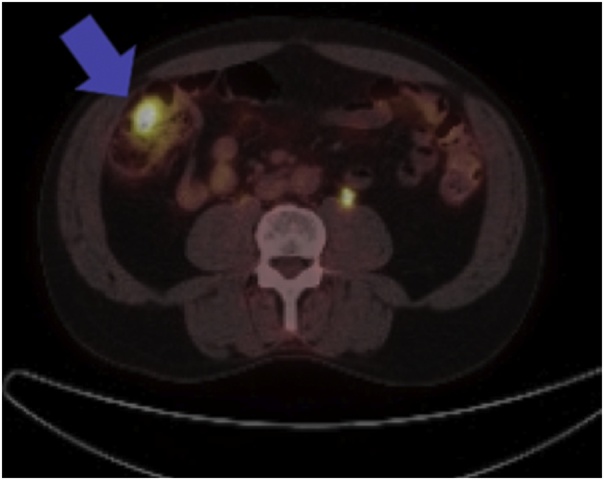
PET-CT scan of the chest, abdomen, and pelvis. Axial slice of the same PET-CT scan demonstrating the same hypermetabolic focus in the cecum (demonstrated by the blue arrow).
